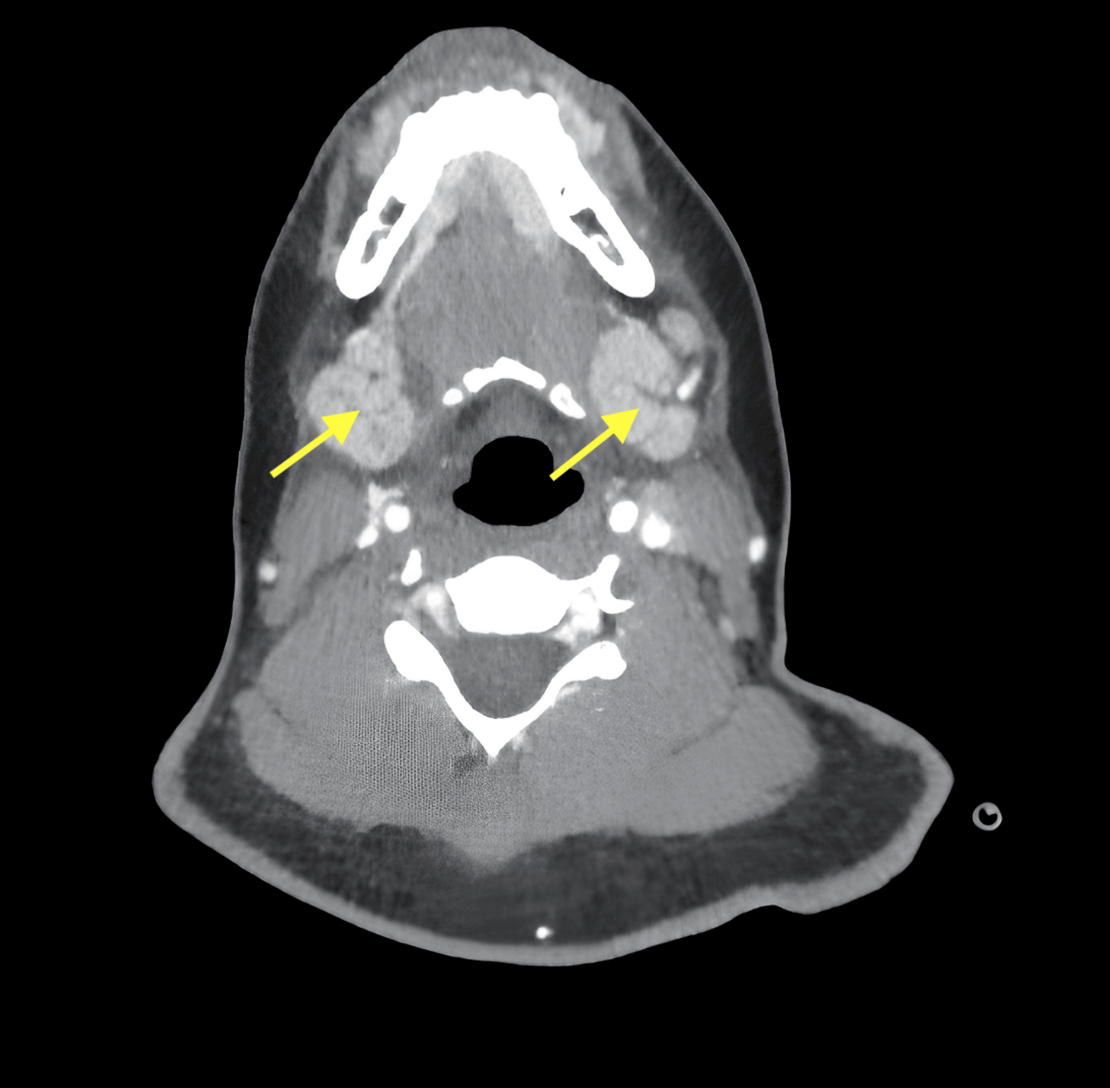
Axial CT neck with contrast Yellow arrows indicate the inflamed submandibular glands.
